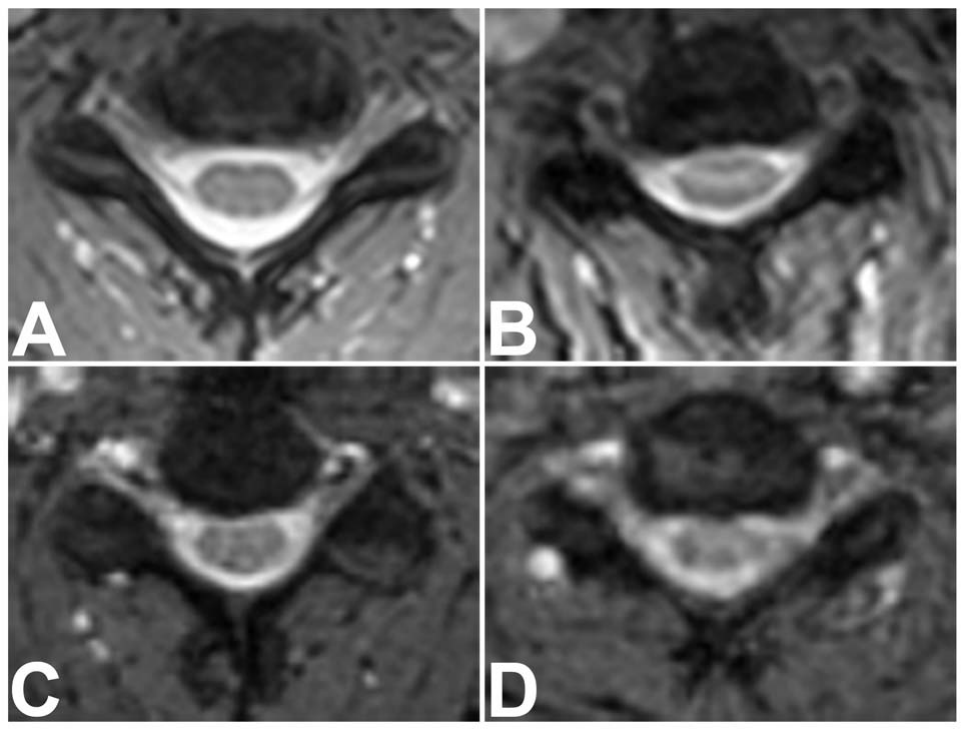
Representative Examples of Image Quality on Axial T2-Weighted Sequences of the Cervical Spine. Axial T2-weighted medic sequences of the cervical spine of four patients, representing the range of qualitative image quality scores obtained with short-bore (A, B) and open MR imaging (C, D). Overall image quality ratings were: “optimal” for sequence A,“good” for sequence B, “moderate” for C, and “poor” for D. No sequence was rated very poor (nondiagnostic).
